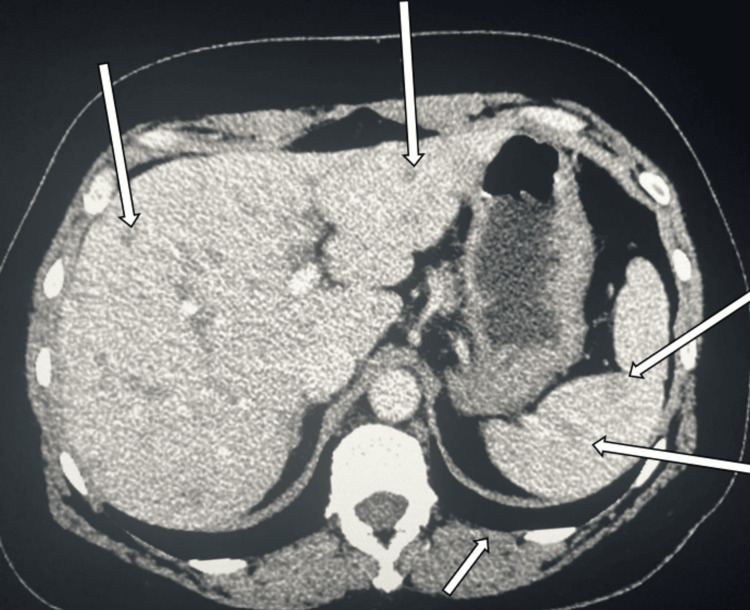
Repeat abdominal CT scan showing stable nodules of the liver and spleen (white arrows) CT: computed tomography
